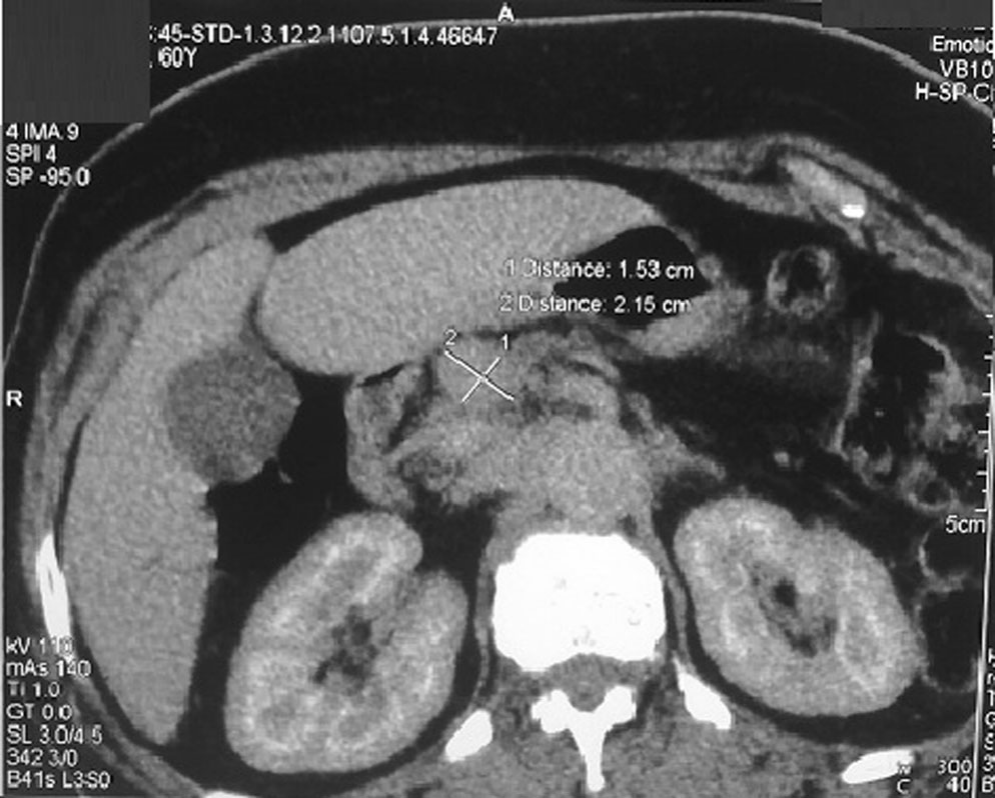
Computed tomography: limited tissue mass in the pancreatic body, measuring 2.1 × 1.5 cm
